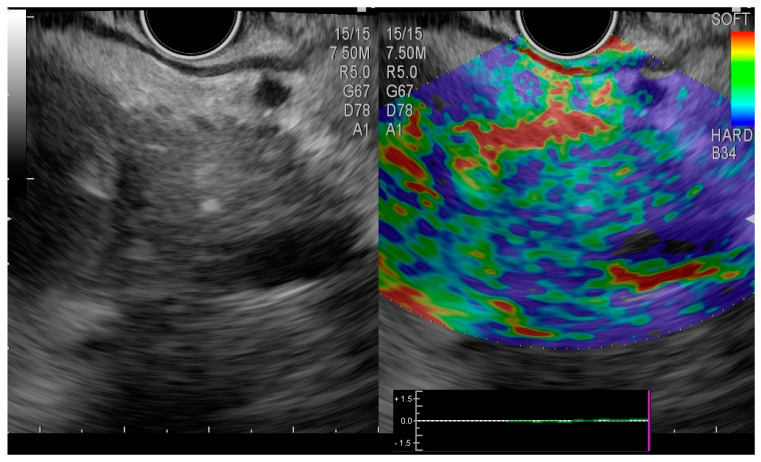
Endoscopic ultrasound elastography. Hypoechoic cephalic pancreatic lesion with mixed pattern.
